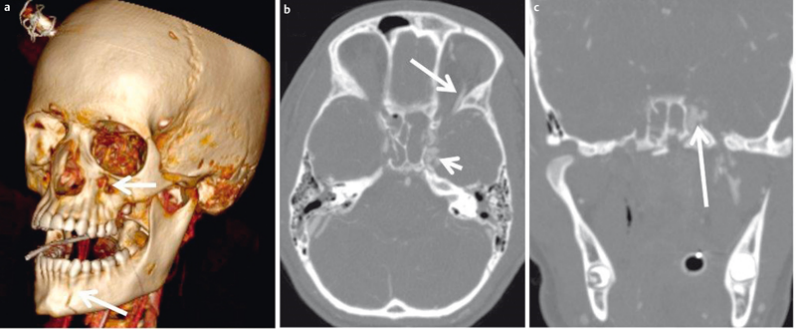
16-year-old patient with severe trauma to the head and progressive exophthalmos and chemosis. a) 3D-VRT reconstruction demonstrates the extent of the fracture with only minimal displacement of the fragments. b) Contrast-enhanced CT angiography demonstrates opacification of the cavernous sinus (short arrow) and the superior ophthalmic vein (long arrow) indicating carotid cavernous sinus fistula. c) Coronal MIP reconstruction (maximum intensity projection, MIP) demonstrates the fistula (arrow).
